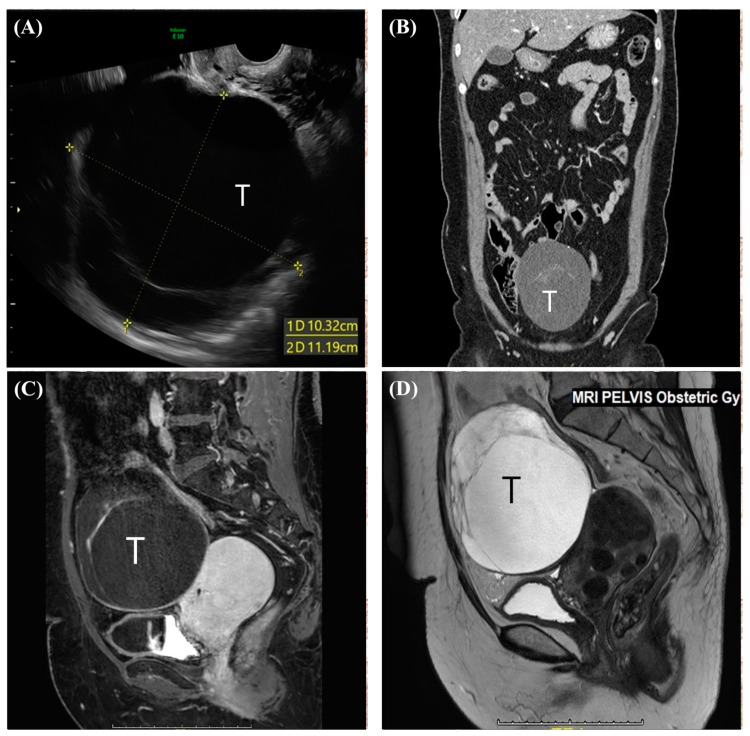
(A) Transvaginal ultrasonographic image of pelvic cavity showing a right adnexal cystic mass with septation. (B) Contrast-enhanced CT in the delayed phase (coronary view) showing a 12 cm abdominopelvic cystic mass with a thick septum which located abdominopelvic cavity. (C) T1-weighted magnetic resonance image (Sagittal view) with tumor (T) showing slightly hypointense compared to skeletal muscle and enhanced by gadolinium at septum. (D) T2-weighted magnetic resonance image (Sagittal view) with tumor (T) showing a hyperintense mass with a curvilinear dark signal intensity.
